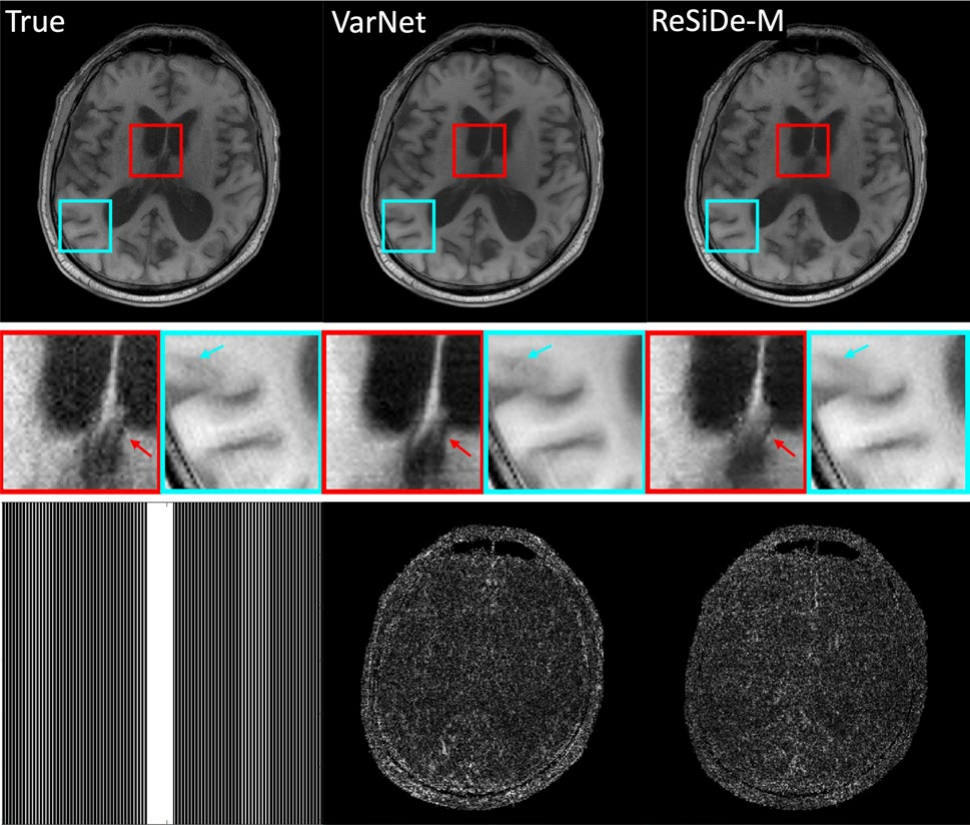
An example showing reconstruction of a T1-weighted image with equispaced (ES) sampling. To highlight differences, the second row magnifies two areas in the brain. The arrows point to features where the two reconstructions show subtle differences. The third row shows the sampling mask (left) and the absolute error maps after five-fold amplification
